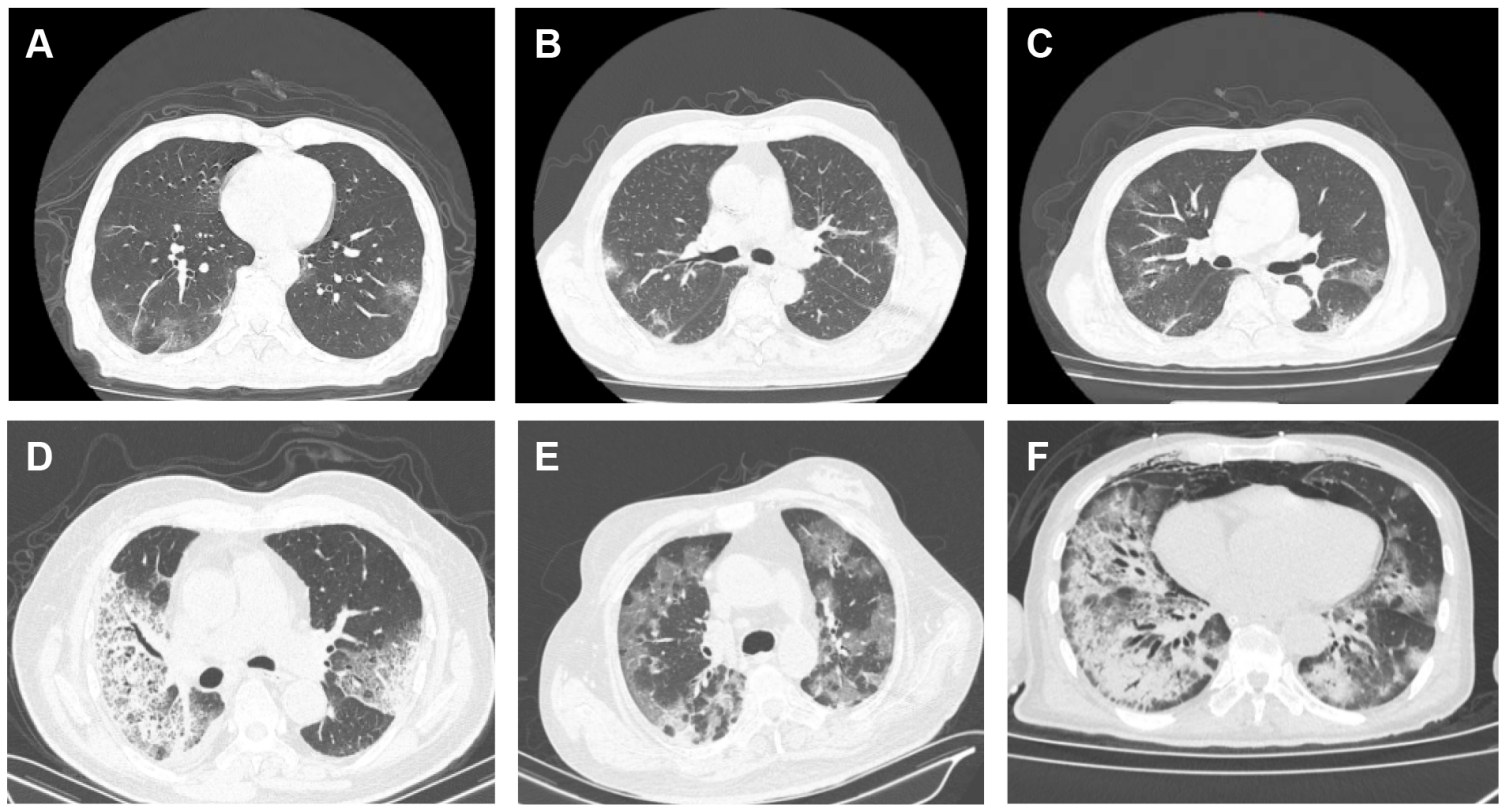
Chest CT images of patients with infection of the novel coronavirus Omicron variant. (A–C) Non-severe cases showing multiple patchy ground-glass opacities and high-density lesions with unclear borders in both lower lobes: (A) A 62-year-old male with fever, cough, and sputum for 12 days; (B) A 26-year-old female with fever for 6 days; (C) An 89-year-old male with fever for 7 days. (D, E) Severe cases: (D) An 81-year-old male with fever for 7 days, showing multiple patchy high-density lesions in both lungs; (E) A 59-year-old female with fever and dyspnea for 7 days, showing multiple patchy high-density lesions and thickened interlobular septa in both lungs; (F) A 64-year-old male with fever for 13 days, showing multiple patchy high-density and ground-glass opacities with blurred margins in both lungs.
